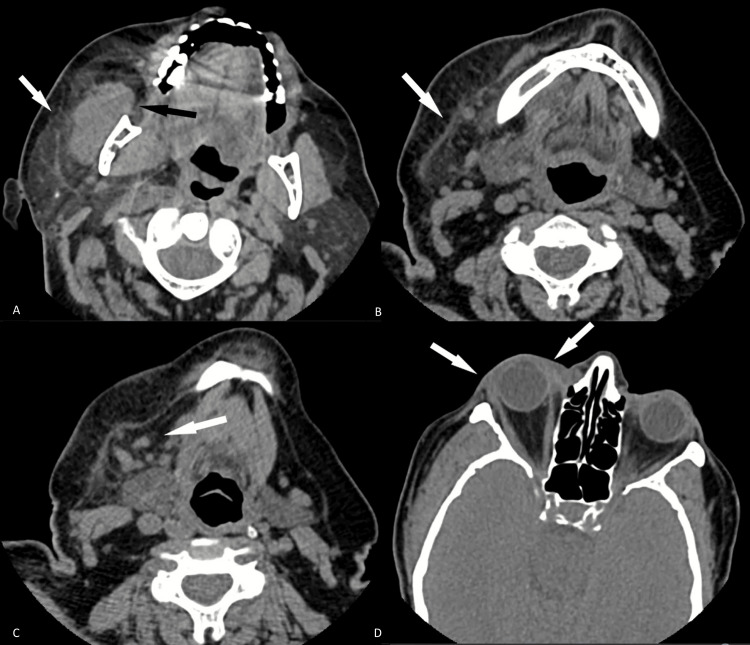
CT images of the maxillofacial skeleton and orbit showing findings of right-sided acute suppurative parotitis and orbital cellulitis. Axial CT of the maxillofacial skeleton shows asymmetric enlargement of the parotid gland with increased adjacent fat stranding consistent with inflammatory changes (white arrow, A) and acute right suppurative parotitis. There is also asymmetric enlargement of the right masseter (black arrow, A) and platysma muscles (white arrow, B) with numerous prominent right level 1B cervical lymph nodes (white arrow, C). Axial CT of the orbit demonstrates increased soft tissue edema and fat stranding within the extraconal palpebrae tissues spanning from the medial to lateral epicanthal folds (white arrows, D) concerning for orbital cellulitis. There was no retrobulbar extension.
